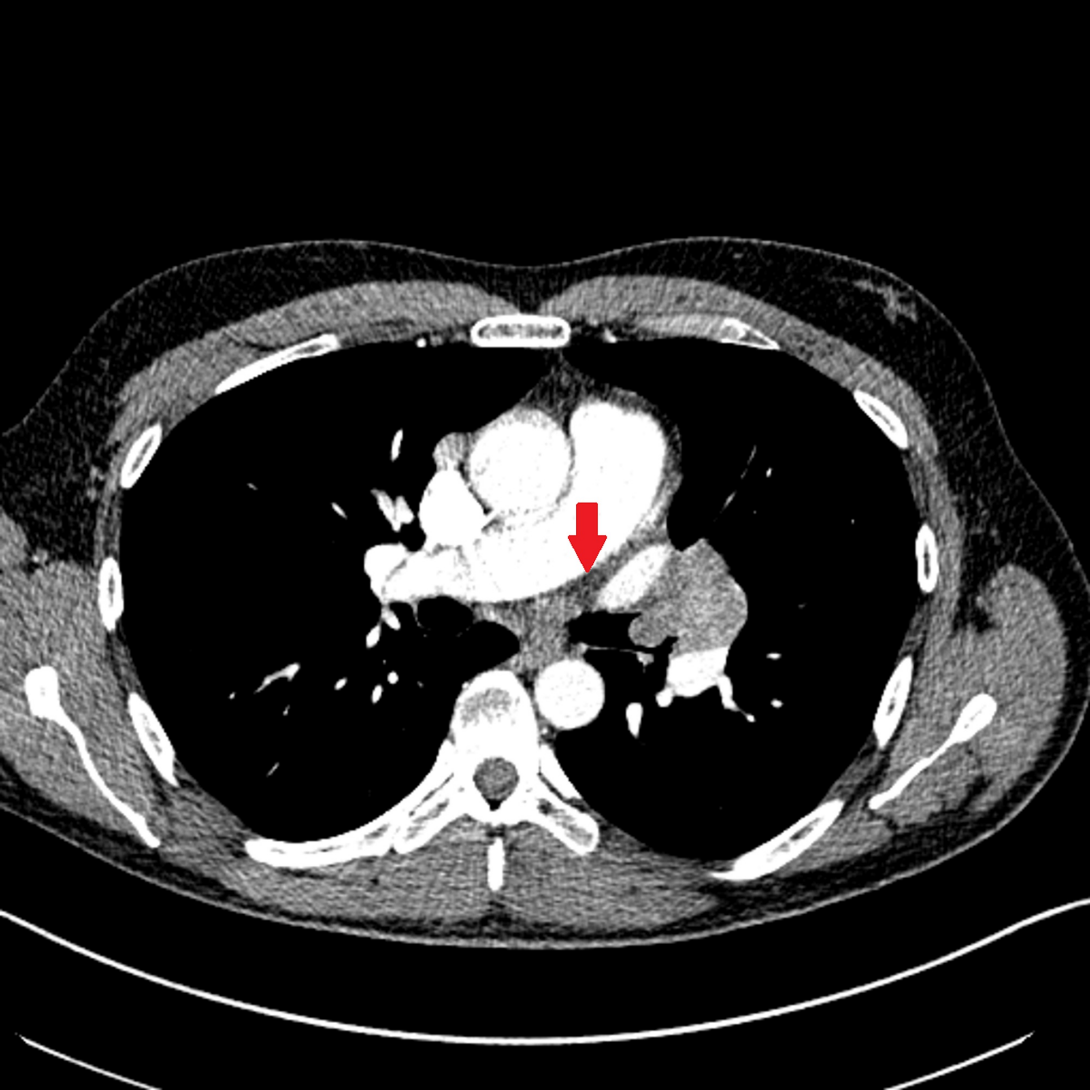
Chest computed tomography Chest computed tomography showing endobronchial mass compromising left upper lobe (red arrow).
